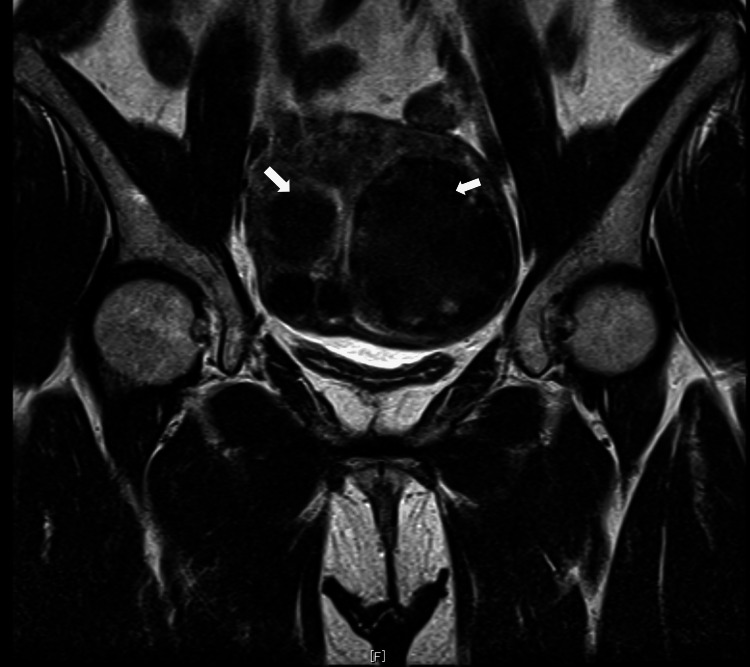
MRI (T2-weighted image, coronal section). Only multiple uterine fibroids are visible.
